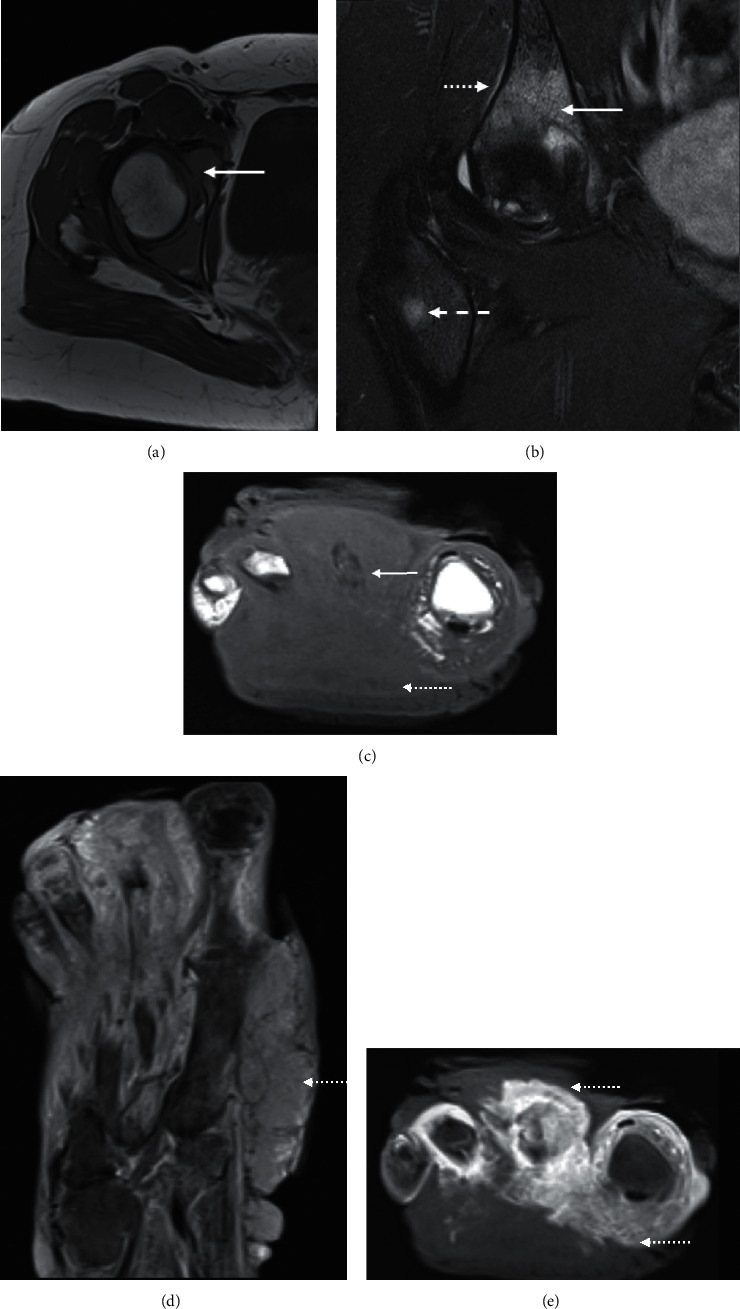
Metastases. Axial T1 (a) pelvis at the level of the hip joint of a 47-year-old female with metastatic breast cancer involving the right acetabulum. Ill-defined acetabular cortex is consistent with cortical breach of tumour and intraarticular extension (arrow). Tumour exhibits mass-effect that is usually readily apparent on MRI. Tumour is usually hyperintense in fluid-sensitive sequences which can mimic infection. Coronal PDFS (b) at the anterior acetabular margin with fluid-hyperintensity of the tumour breaching the acetabular cortex (arrow). Periosteal extension lateral pelvis (dotted arrow). A separate metastatic deposit also presents in the proximal right femoral shaft which helps clinch the diagnosis (dash). Axial T1 (c) and coronal STIR (d) and axial fat-saturated postcontrast T1 (e) of the right foot of a 38-year-old man with AIDS-related Kaposi sarcoma. The fungating, partially necrotic/enhancing mass (e) is centred in the forefoot, particularly involving the second metatarsal (arrow). The signal intensity of the involved bone mimics that of osteomyelitis, although infection should not be infiltrative and mass-forming (dotted arrows).
